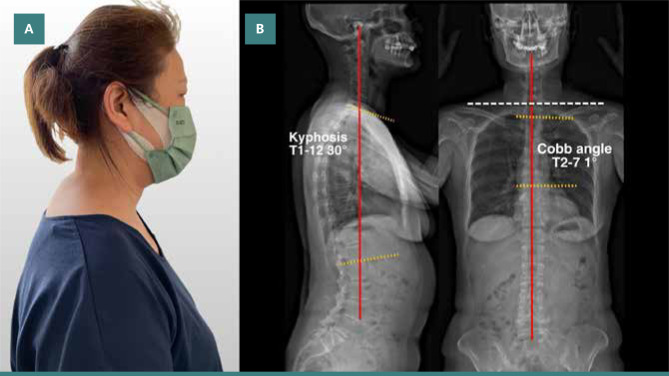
A: During re-evaluation 9 months later, the size of the hump was diminished after treatment. B: EOS® radiography showed posture balance with improved shoulder balance, central sacral vertical line, gravity line, kyphosis angle, and scoliosis measurement of Cobb angle. The kyphosis reduced from 45° to 30° and the Cobb angle reduced from 9° to 1°
